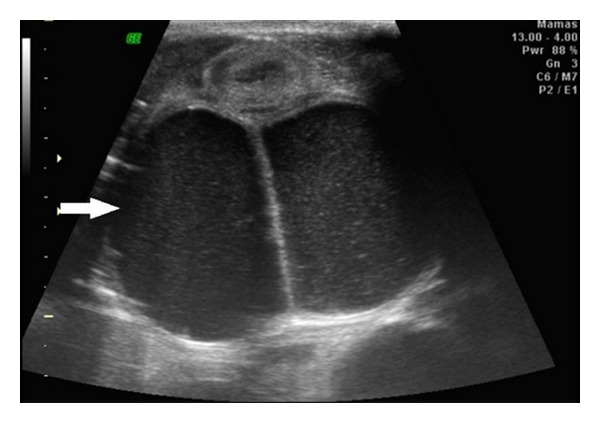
Ultrasound scan after the bladder was completely empty, shows the septated cystic mass (white arrow) occupying the region of the newborn's uterus.
